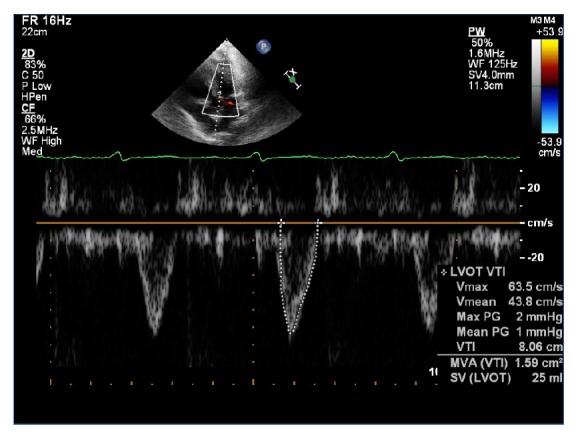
At the time of clinical deterioration, pulse Doppler of the LVOT and continuity equation calculated SV/CO (LVOT dimension-2.0 cm, VTI-8.1 cm) suggested low stroke volume (25 ml) and cardiac output (2.22 L/min).
